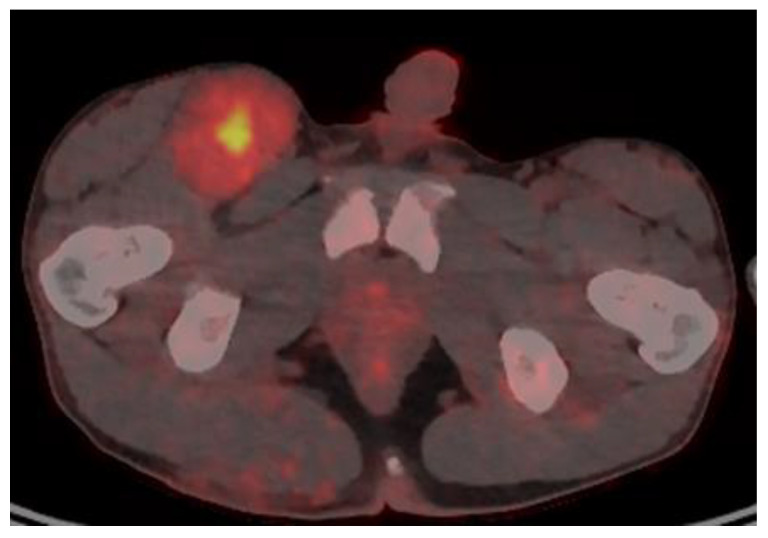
18F-FDG PET/CT of a patient with NF1 and an MPNST of the right groin, with a maximal SUV of 7.
